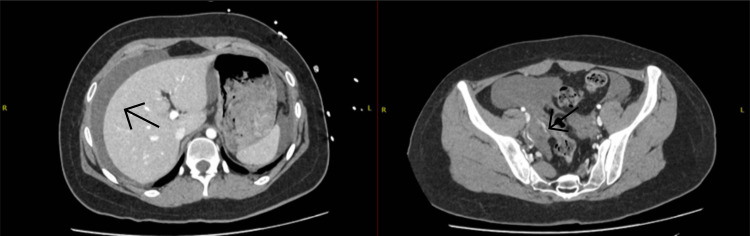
A CT of the abdomen and pelvis shows hemoperitoneum and an area of concern: ongoing bleeding in the right ovary.
